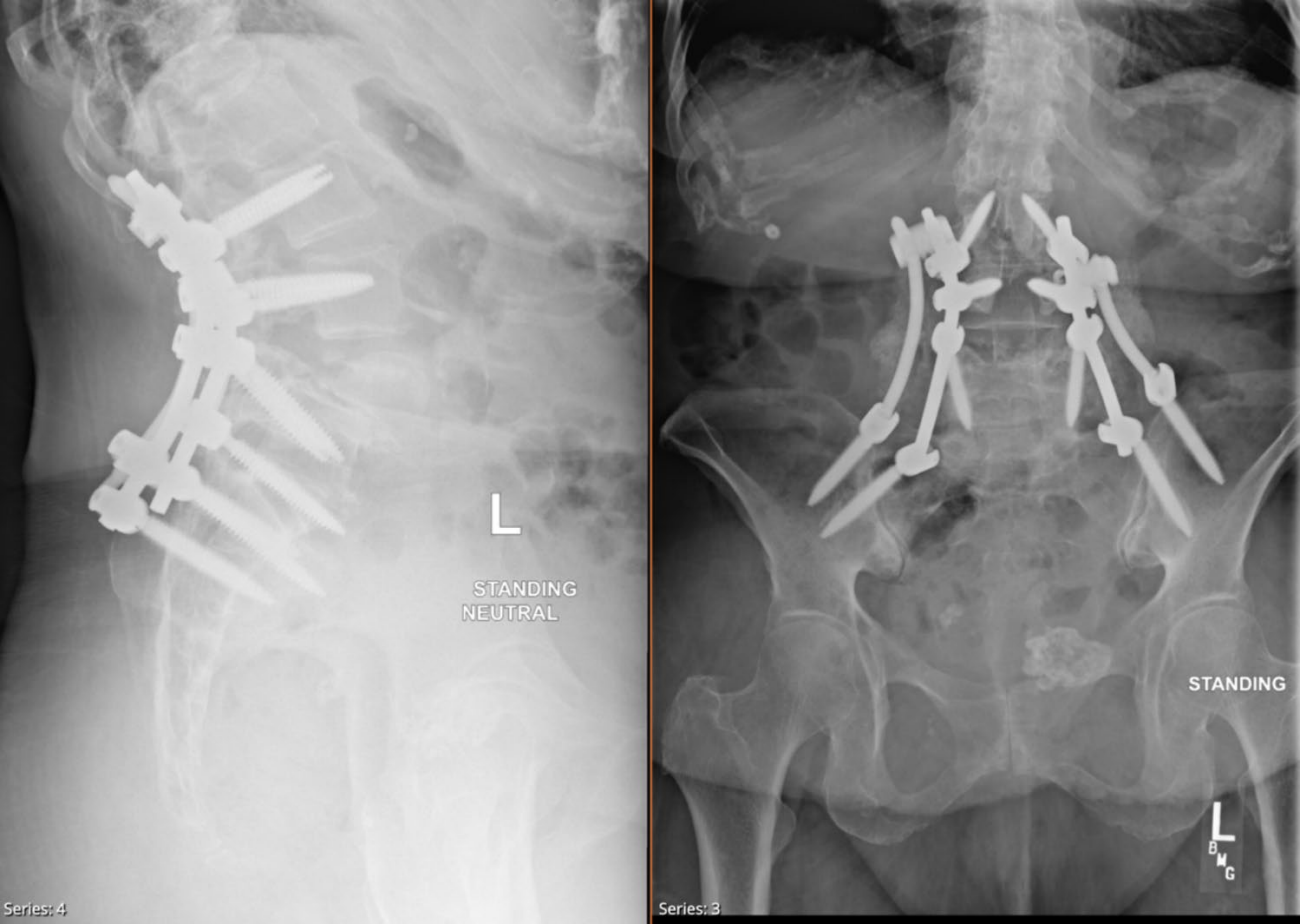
Lumbar spine radiographs (AP and Lateral) at final follow-up after L2–pelvis posterior spinal fusion with revision L3–L4 laminectomy and L4 pedicle subtraction osteotomy
